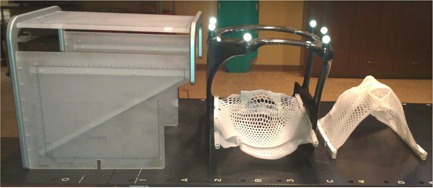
(left) CT localizer, (middle) infrared frame, (middle and right) lower and upper components of the head and neck Brainlab mask.
